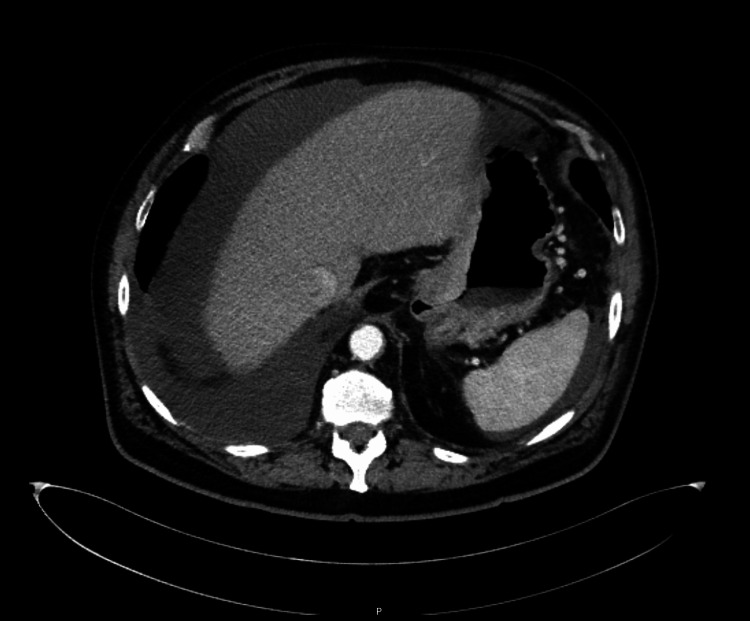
Abdominal CT scan showing marked thickening in the cardia and the region of the gastric, as well as large-volume ascites.
